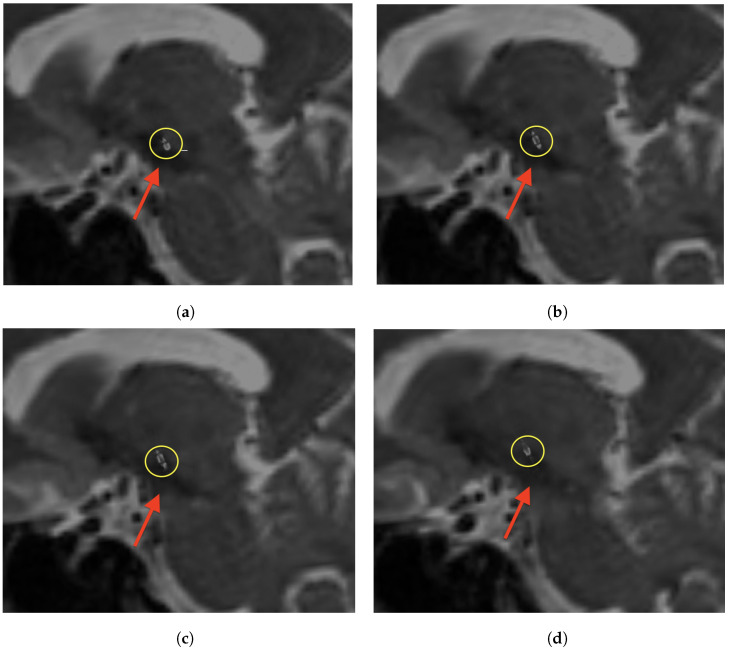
Sagittal non-contrast T2-weighted MRI with DynaCT superimposed using alpha blending. The subthalamic nucleus is displayed as a T2-hypointense streak (red arrow). All parts of the electrode that are relevant for optimizing the stimulation can be clearly delineated (yellow circle). The image sequence transitions from medial (a) to lateral (d). (a) Proximal circular contact within STN. (b) Proximal segmented contacts for steering the electric field. (c) Distal segmented contact. (d) Distal circular contact.
